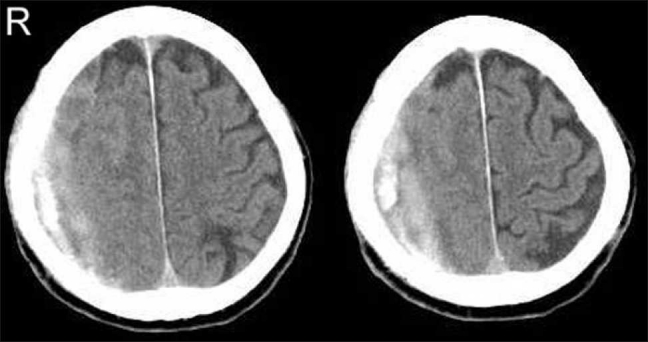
CT image of case 2 obtained after the MMA angiography procedure, showing leakage of contrast enhancement into the hematoma cavity
